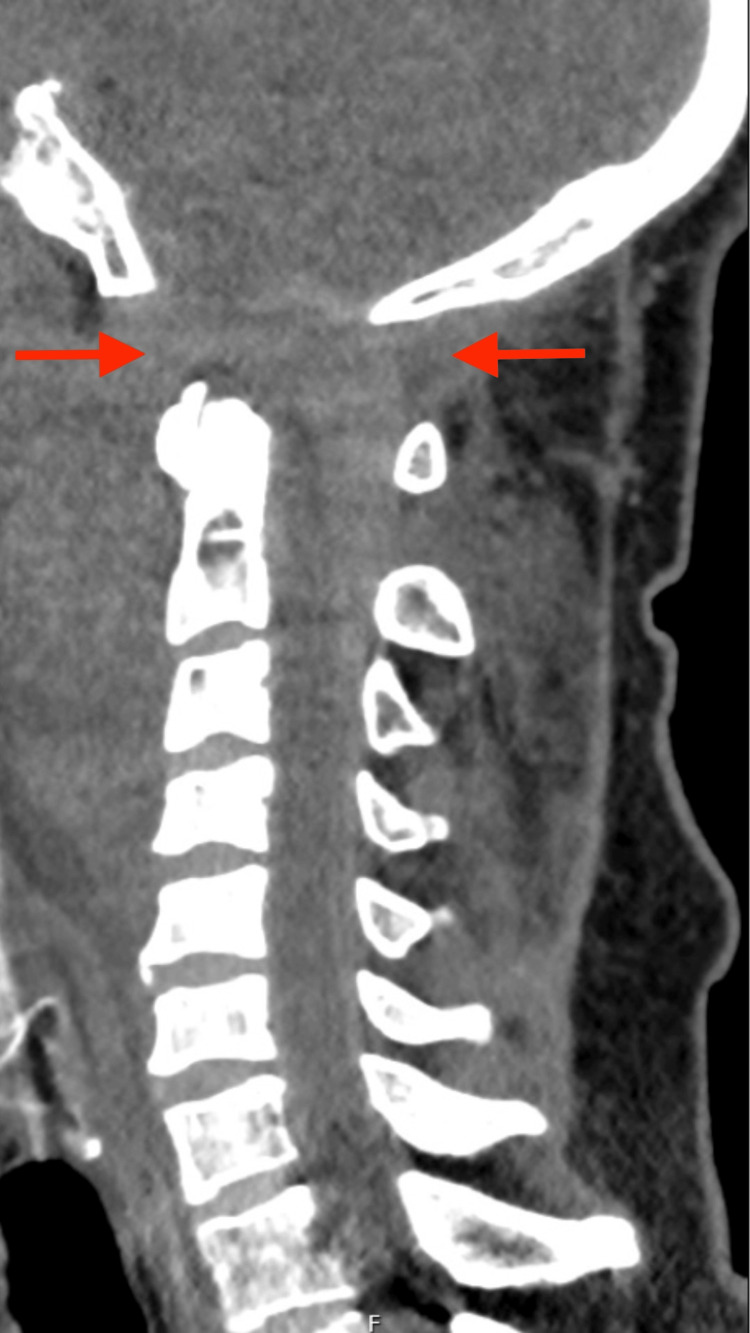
Sagittal computed tomography (CT) reconstruction showing widening of the occiput–C1 joint space (arrows), consistent with atlanto-occipital dissociation.
